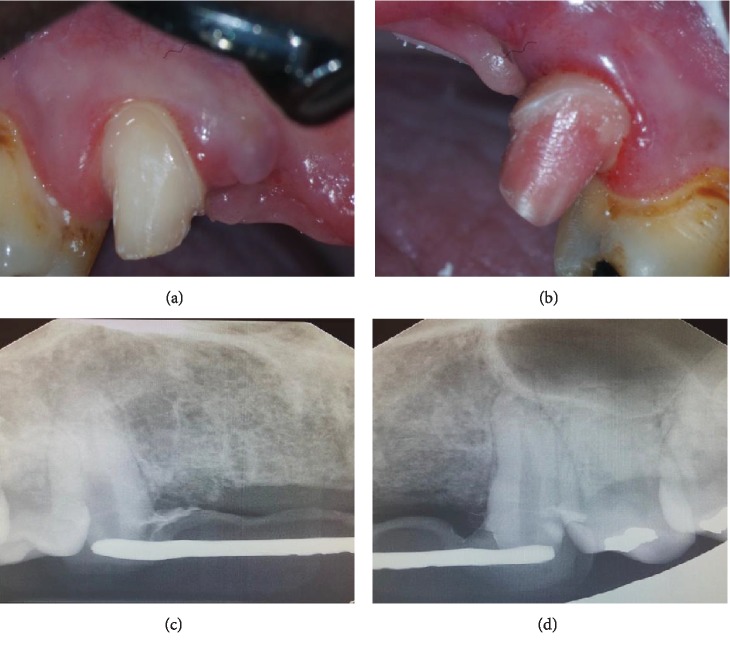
(a) Clinical photograph of tooth #6 after the removal of provisional FPD, normal clinical appearance. (b) Clinical photograph of tooth #11 after the removal of provisional FPD, red hue appearance. (c) Radiograph of tooth #6 before the removal of provisional FPD. (d) Radiograph of tooth #11 before the removal of provisional FPD.
